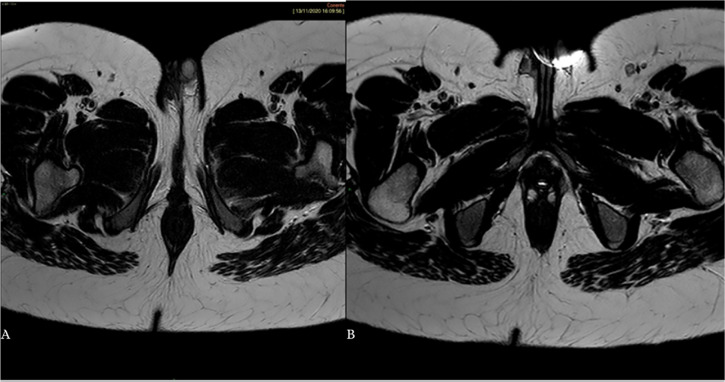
T2-weighted axial image: left (A) and right (B) testicles. Bilateral
